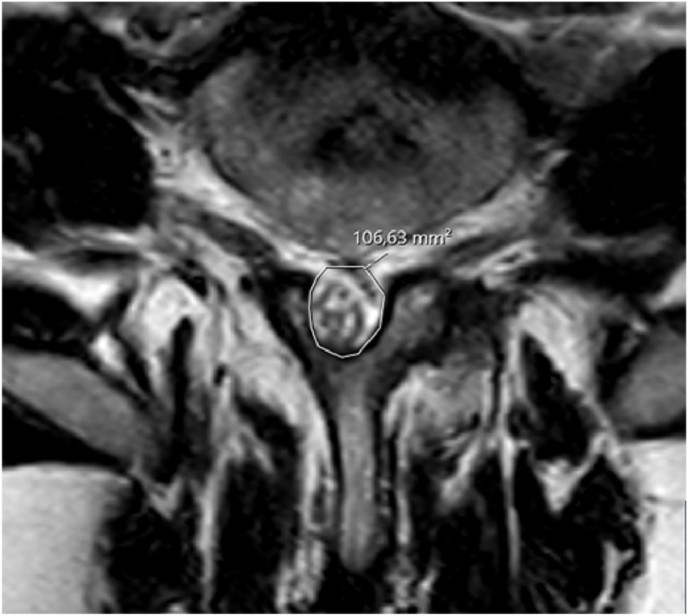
Axial T2-weighted MRI scan demonstrating the dural sac cross-section areal (DSCA). The white line indicates the outline of the DSCA, which is 106.63 mm^2^.
